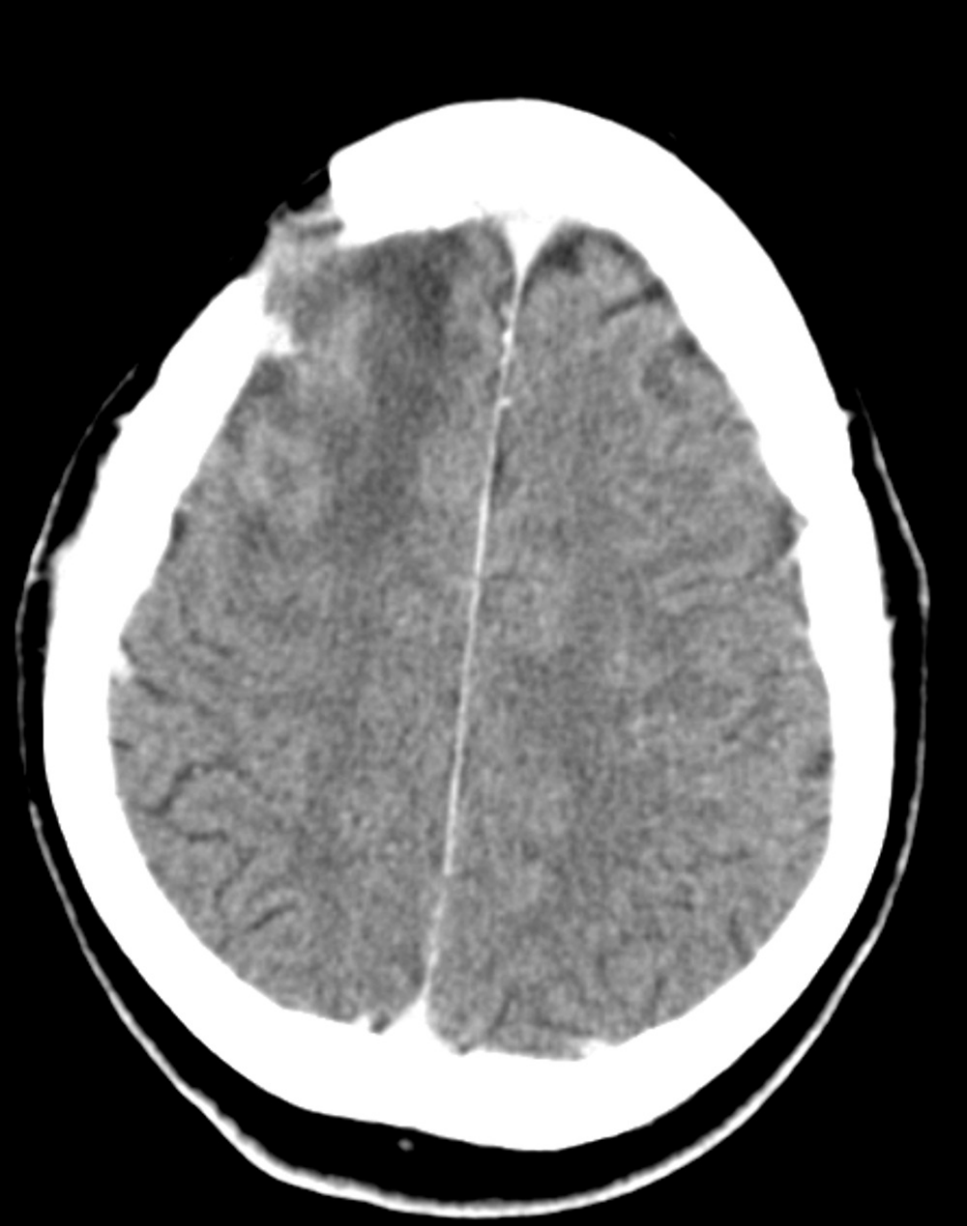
Delayed CT head with contrast showing resolution of abscess
